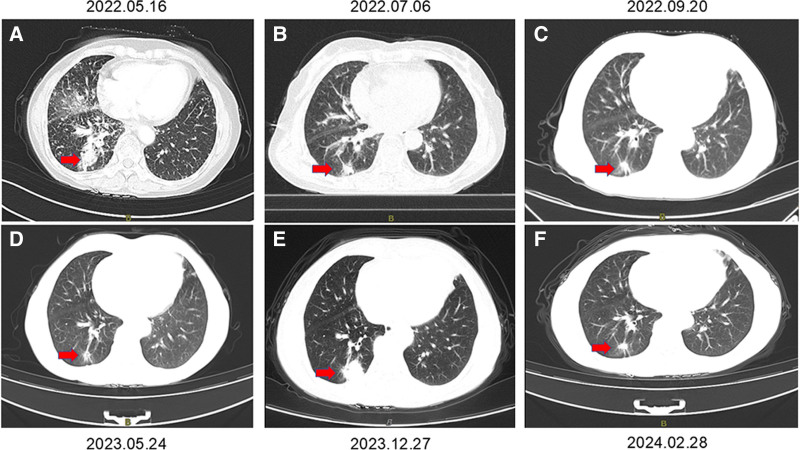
Representative CT Images at Different Time Points. The CT scan shows a lesion in the lower right lung, with the lesion indicated by a red arrow. CT = computed tomography.
